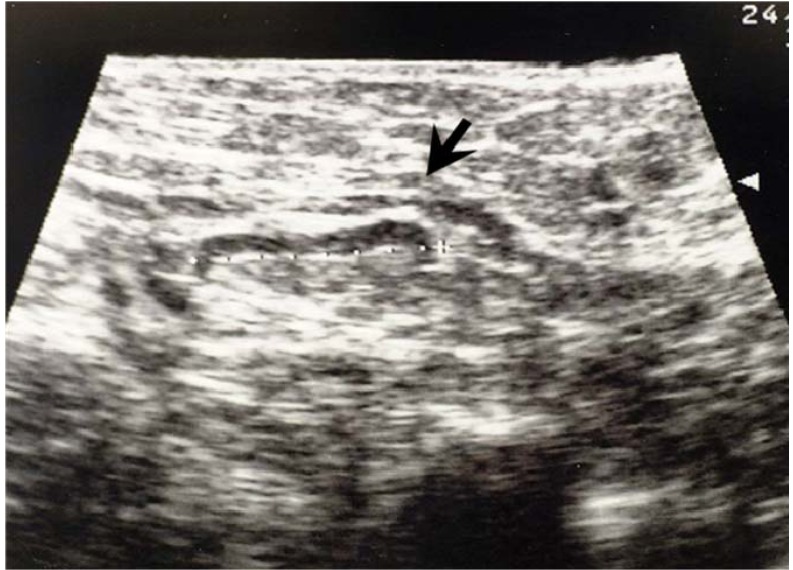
Ultrasonographic appearance of a normal lymph node (type 1). This lymph node (arrow) shows thin C-shaped low echoic cortex and regular cortex.
